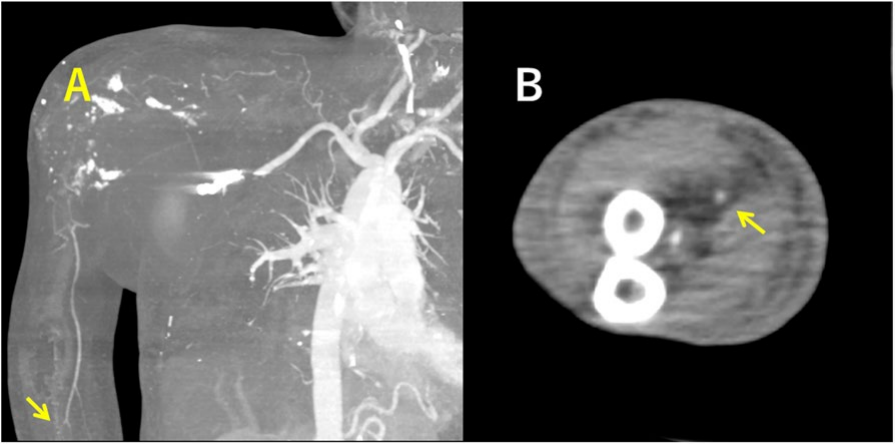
Presence of blood-flow at the right radial artery (arrow) on the maximum intensity projection of computed tomography angiography (A) and on the axial view of contrast-enhanced computed tomography (B)
